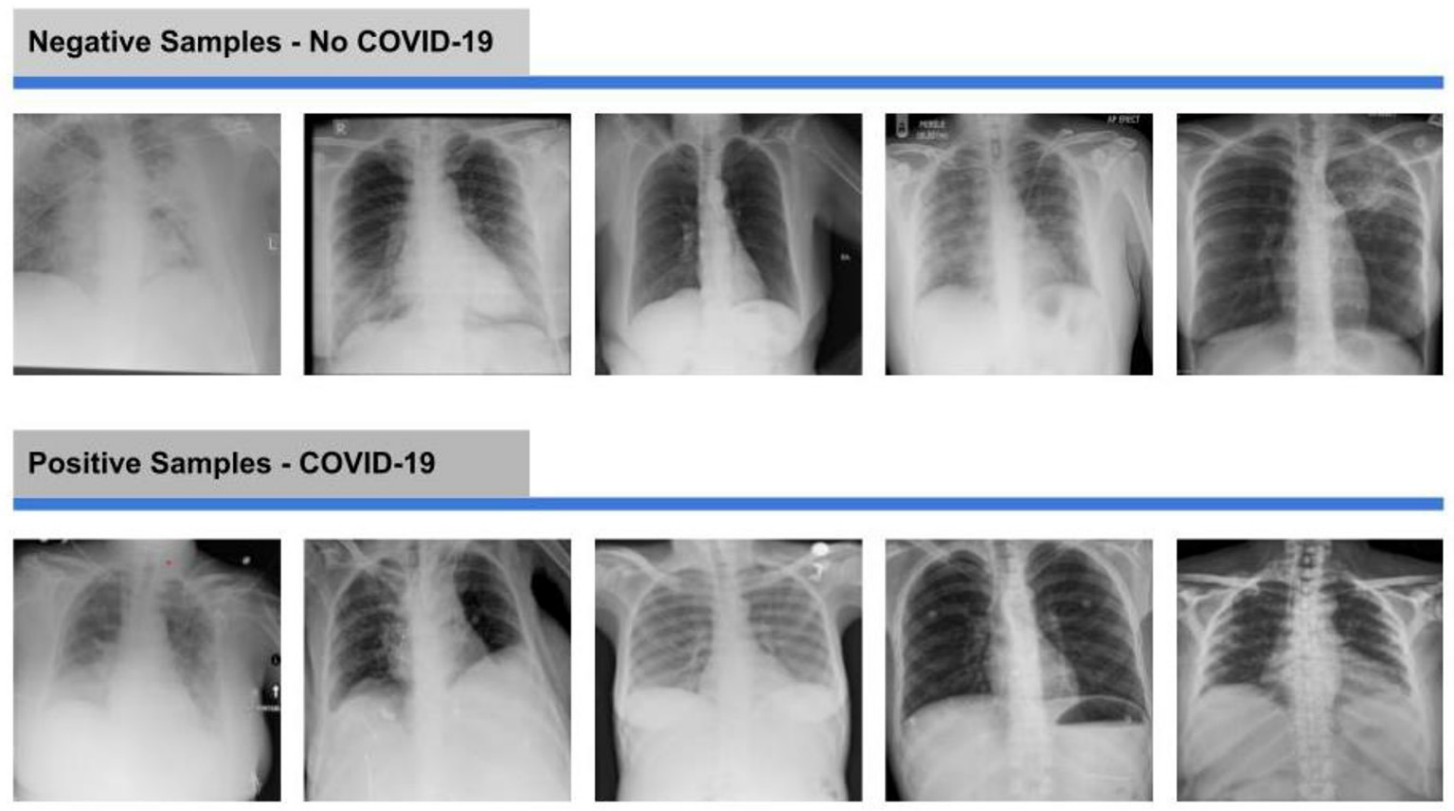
Negative and positive samples of X-ray images from the dataset.
